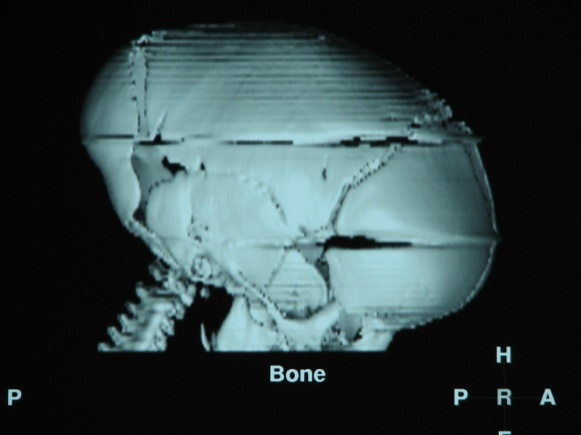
Postoperatory 3D CT in scaphocephaly
